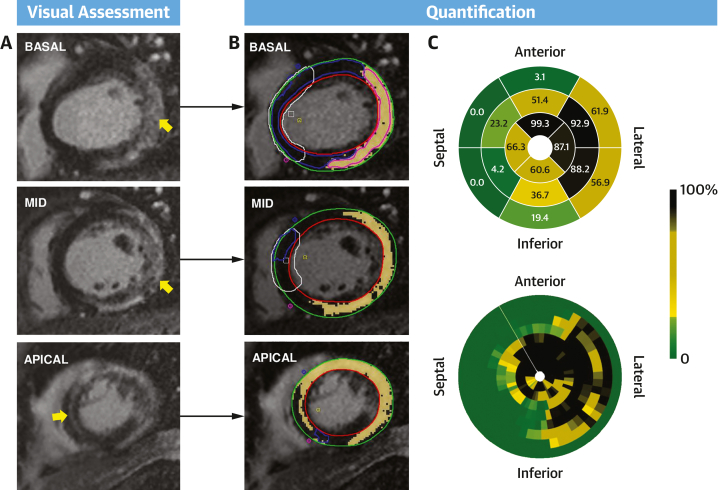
Cardiac Magnetic Resonance in Nonischemic Cardiomyopathy (A) Late gadolinium enhancement cardiovascular magnetic resonance images were visually assessed to determine whether myocardial fibrosis (MF) was present or absent. If MF was present (appears white on late gadolinium enhancement), quantification was undertaken. (B) To this end, epicardial and endocardial contours (green and red, respectively) were semiautomatically delineated. Total fibrosis and gray zone mass were quantified using various signal thresholding methods. In this example, the basal segments showed extensive, heterogeneous MF (yellow arrows) in a noncoronary distribution over the left ventricular free wall, with a distinct epicardial and midmyocardial distribution toward the midventricular and apical segments. (C) The polar maps show the distribution of MF according to the American Heart Association 16-segment model and to smaller segments (100 segments over 8 short-axis slices, starting from the junction of the right ventricular wall and the interventricular septum [white line]). The scale range is from 0 (green, no MF) to 100% (black, entire segment is 100% MF).
